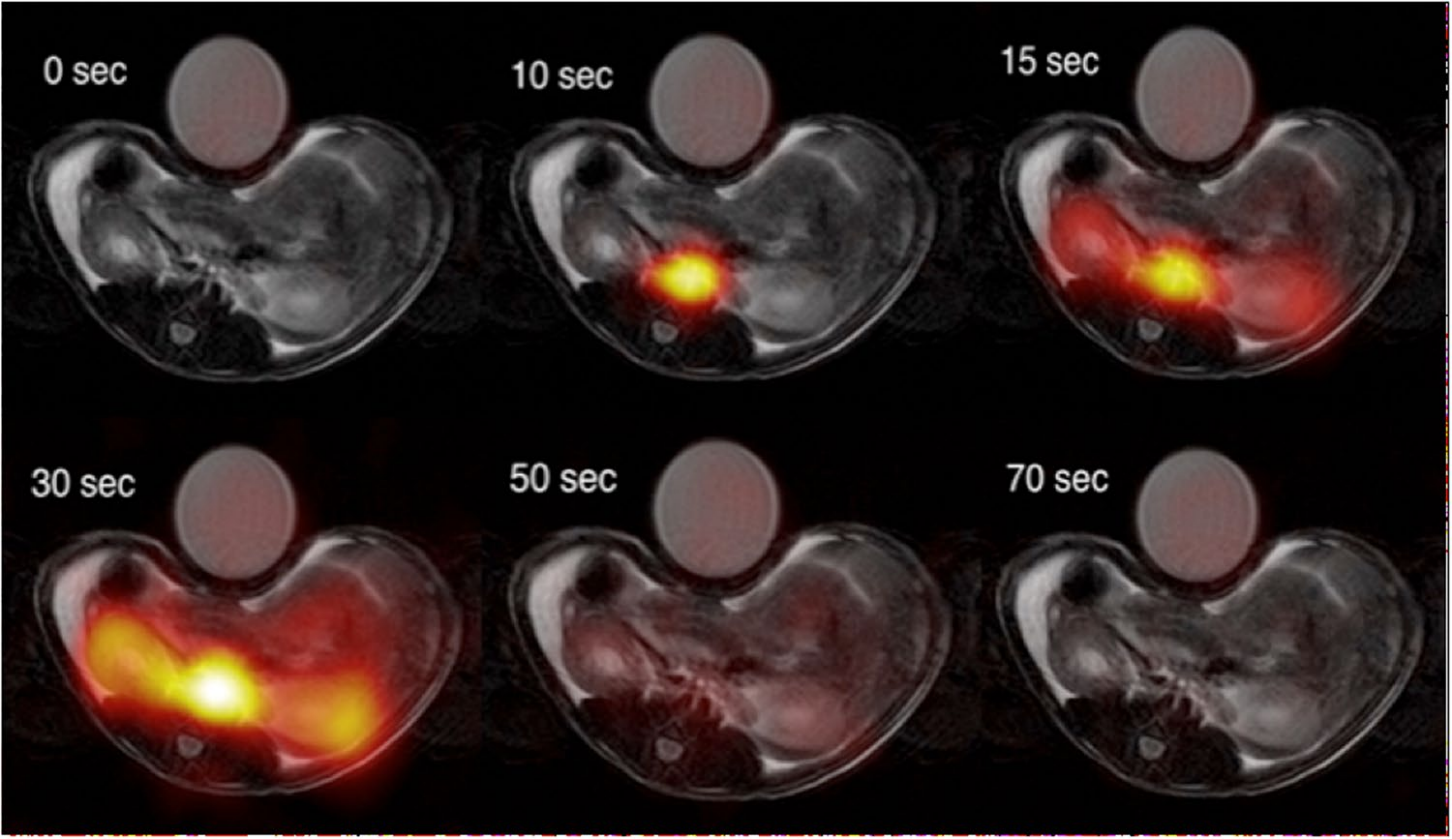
Examples of ^11^C-acetate uptake in the aorta and kidneys over time. Positron emission tomography ^11^C-acetate signal overlaid ^1^H-anatomical MR images of a coronal slice, showing two kidneys and the presence of a signal in the aorta and following the kidneys. MR; magnetic resonance.
